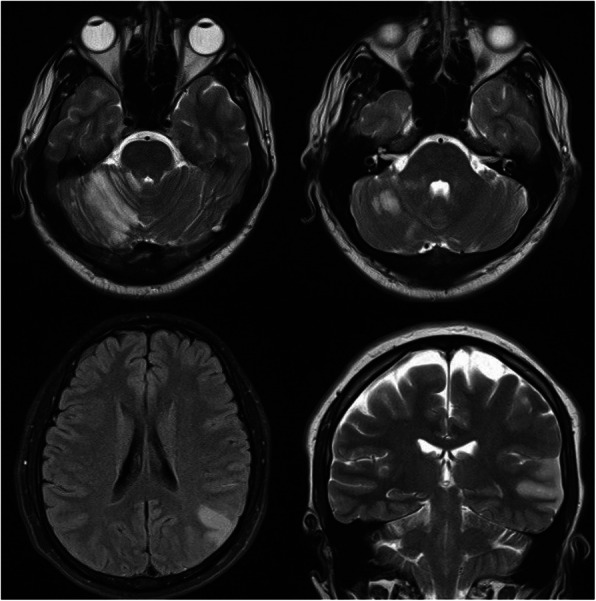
Brain MRI (magnetic resonance imaging) showing multiple cerebellar and parieto-occipital ischemic lesions
